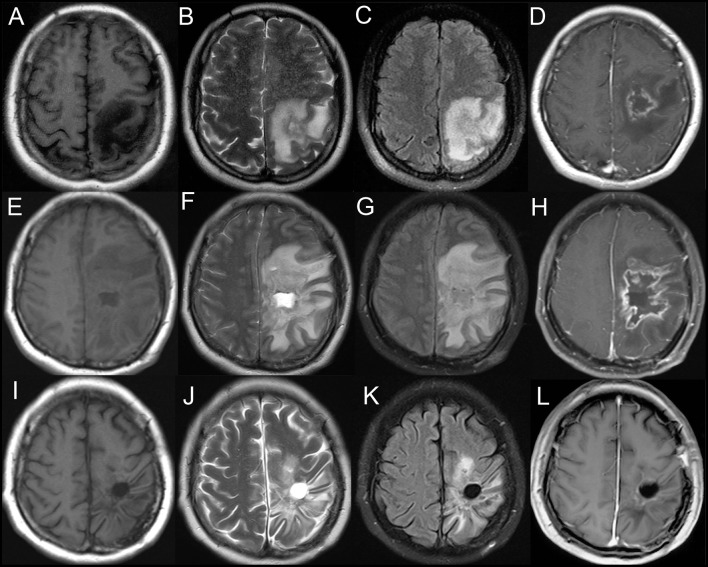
Axial T1-weighted (A), T2-weighted (B), and fluid-attenuated inversion recovery (FLAIR) (C) demonstrating a heterogeneous mass centered in the left parietal lobe. T1-weighted gadolinium-enhanced (D) MRI showing an irregular peripheral enhancement mass with a central non-enhancing area. (E–H) Postoperative MRI scan showing a flaky edema area in the left frontotemporal lobe. Axial T1-weighted (I), T2-weighted (J), fluid-attenuated inversion recovery (FLAIR) (K), and T1-weighted gadolinium-enhanced (L) MRI showing no evidence of contrast enhancement in the left parietal lobe on 6 month follow-up.
